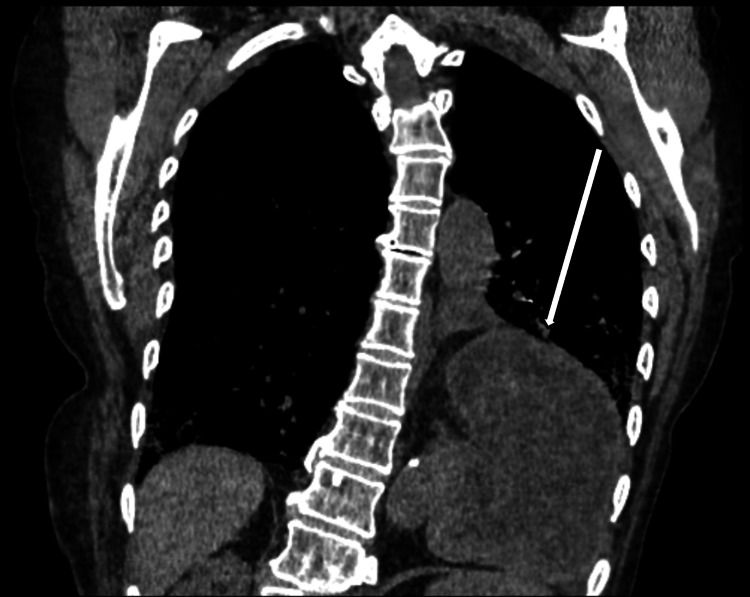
CT Chest Axial view showcasing herniation of the stomach into the left hemithorax.
